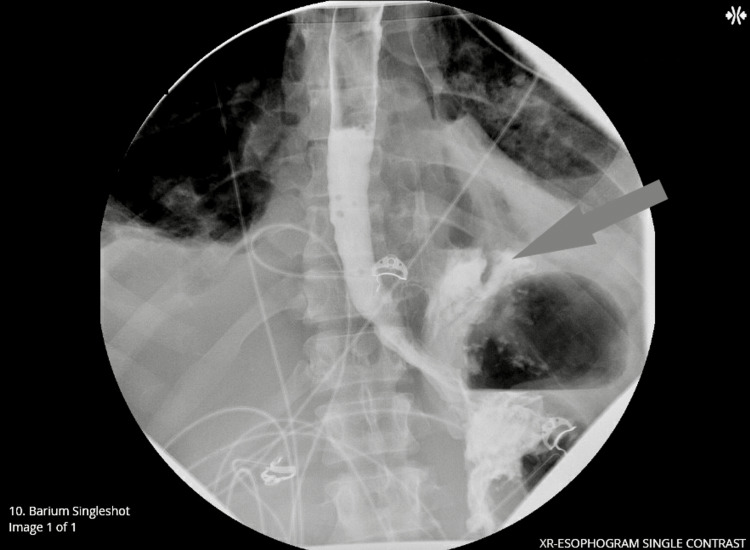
Esophagogram showing contrast leak (arrow) XR: x-ray.
